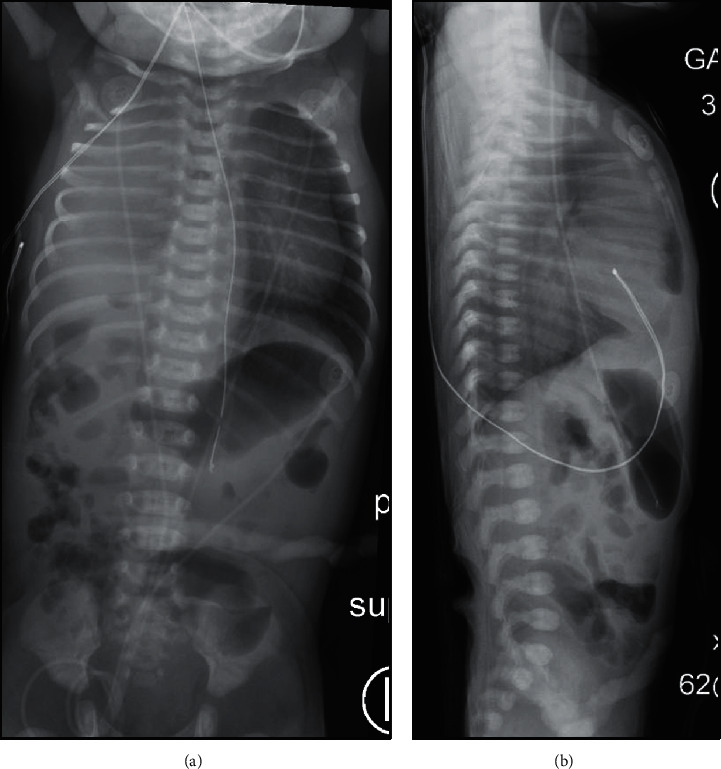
Radiographs upon admission. (a) Anterior-posterior view depicting complete opacification of right hemithorax with leftward mediastinal shift. (b) Cross-table lateral view depicting suspected hernia of abdominal contents into chest cavity.
